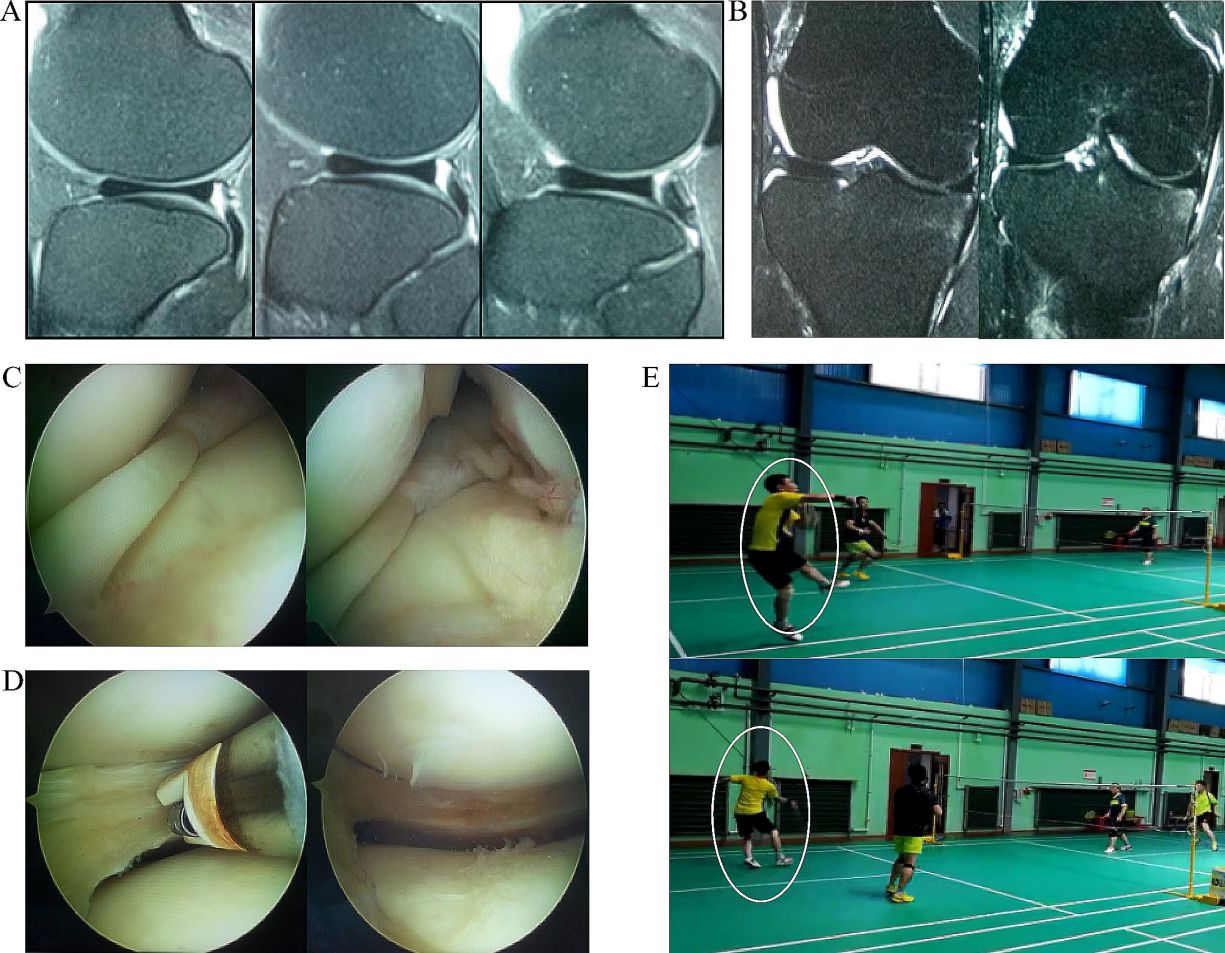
Arthroscopic simple DM plasty for the treatment of lateral DM (male, 52 years old, right knee pain and movement limitation caused by fall during exercise for more than one month. Amateur badminton player). (A) MRI showed that the anterior and posterior corners of more than 3 consecutive sagittal planes were connected. (B) The transverse diameter of lateral meniscus in MRI coronal plane exceeded 20% of the widest transverse diameter of tibial plateau, but the medial edge did not reach the intercondylar eminence. (C) The Watanbe classification of lateral DM is incomplete DM. (D) The part of DM is resected, trimmed and formed under arthroscopy and no meniscus injury was found, moreover, the thickness of meniscus was about 4 mm and the medial edge of meniscus was treated with plasma radiofrequency knife. (E) The patients participating in the badminton match
